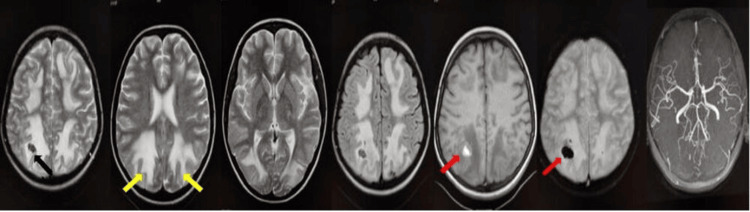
MRI studies of the patient Black arrow: Posterior reversible encephalopathy syndrome with subacute intracerebral hemorrhage; Yellow arrow: Symmetrical periventricular, largely posteriorly located high T2/FLAIR signal is noted; Red arrows: T1 hyperintense focus showing blooming on GRE sequence in right parietal lobe suggestive of hemorrhage FLAIR: Fluid-attenuated inversion recovery, GRE: Gradient recalled echo
